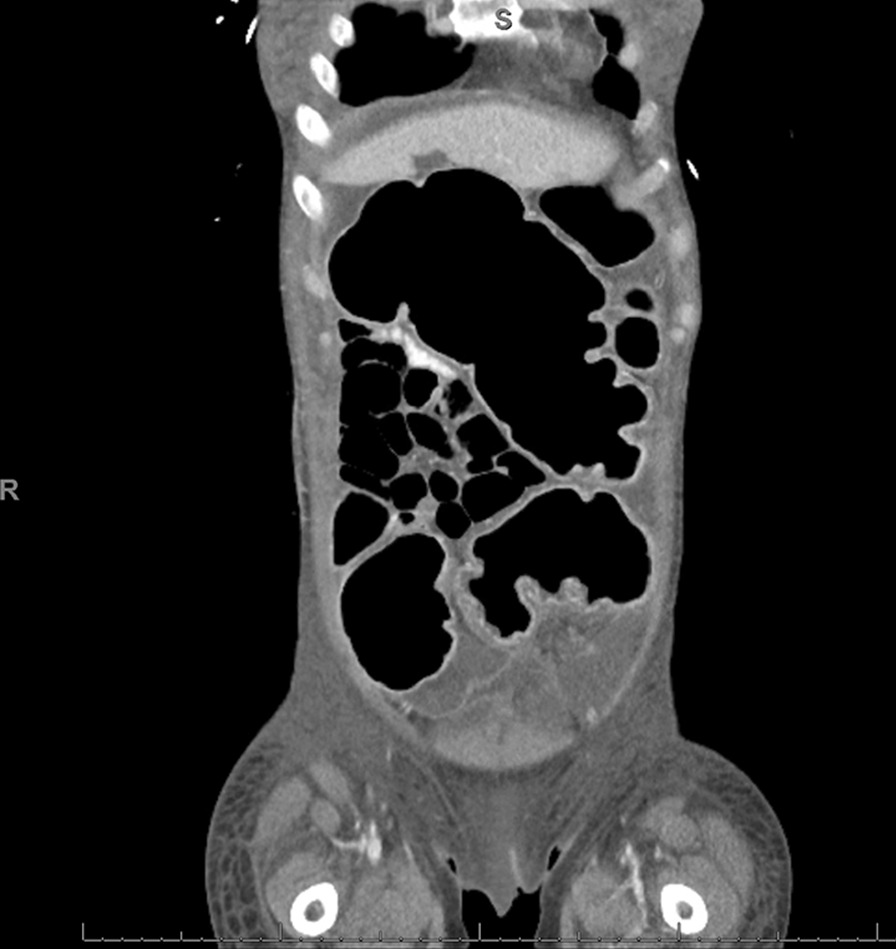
Coronal slice of a computed tomography abdomen/pelvis showing dilated large bowel with partial enhancement of the wall of the colon, suggestive of colitis
